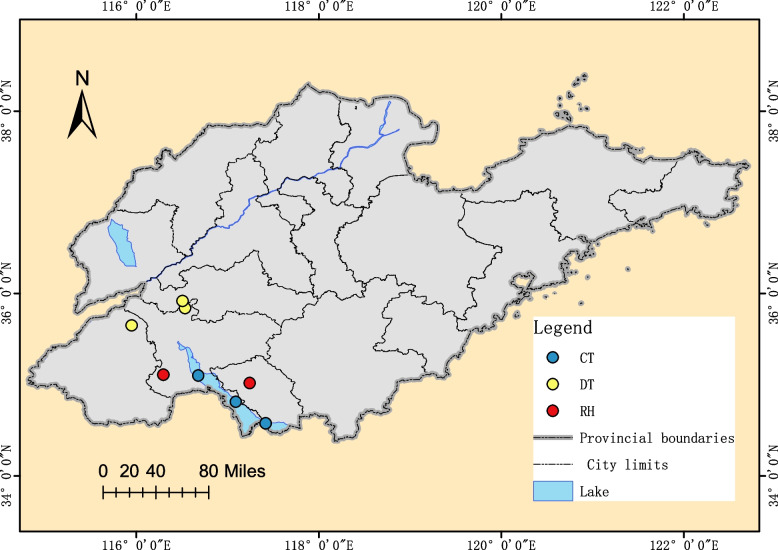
Sampling sites of *Culex tritaeniorhynchus* populations in different ecological environments in Shandong Province, China. CT Lotus Ponds, DT Paddy Fields, RH Irrigation canals
